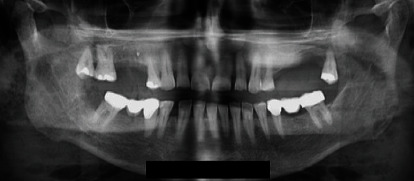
Initial orthopantomography (OPG) X-ray 13/02/2021: partial edentulism; outcomes of conservative and orthodontic therapy, prosthetic rehabilitation.
